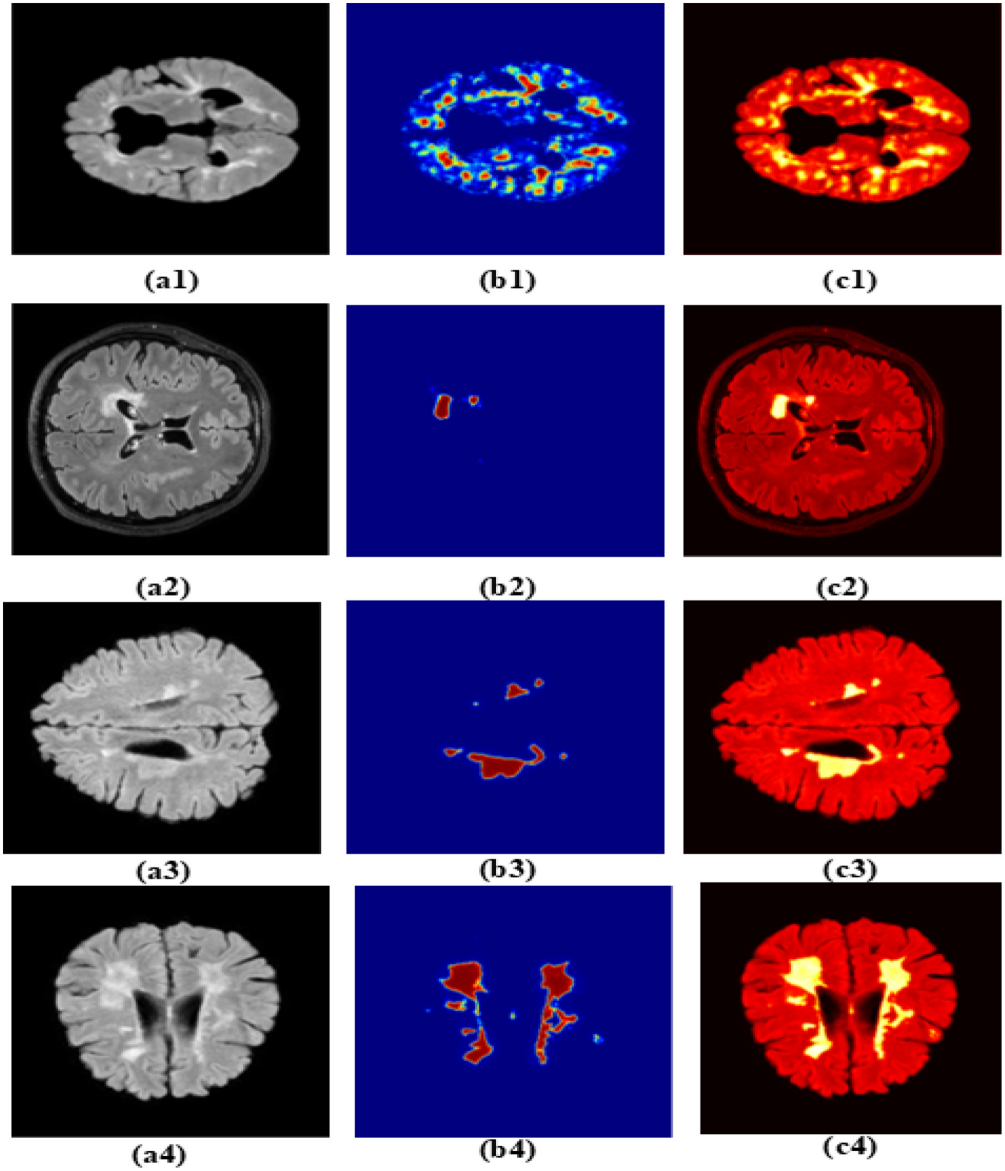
Attention map using MS-DASPNet. (a1–a4) Brain MRI image. (b1–b4) Attention map using GRAD-CAM. (c1–c4) GRAD-CAM overlay.
